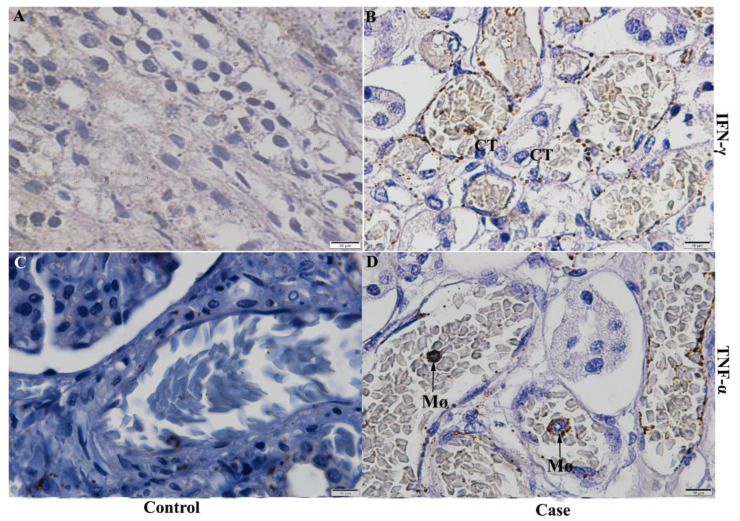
Detection of cytokines in the kidney of fatal cases. (B) Detection of IFN-γ expression around and across collecting tubules in medullary region in Case 1. (D) Detection of TNF-α expression in circulating macrophages in the medullary region in Case 1. (A,C) Control tissue did not show significant detection of these markers. CT—Collecting tubules; MC—Mesangial cells; Mø—Macrophage; Mo—Monocytes EC—Endothelial cells.
